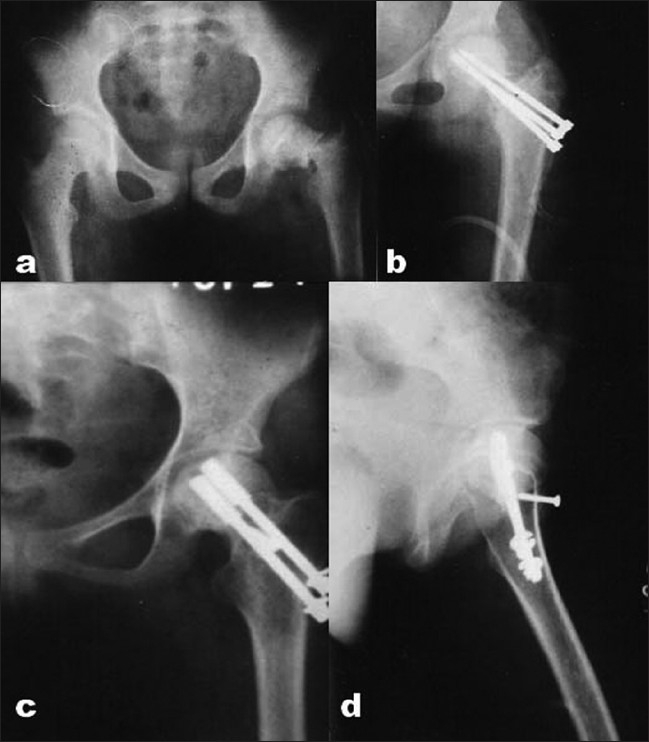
(a) X-ray pelvis with both hip joints (anteroposterior view) showing displaced femoral neck fracture in a male of 9½ years presented after 7 days. (b) An anteroposterior view showing nonunion with failed implants. (c,d) Follow-up radiographs (anteroposterior view and lateral view) at 28 months showing union after Meyer's procedure. The femoral head later on went into AVN
